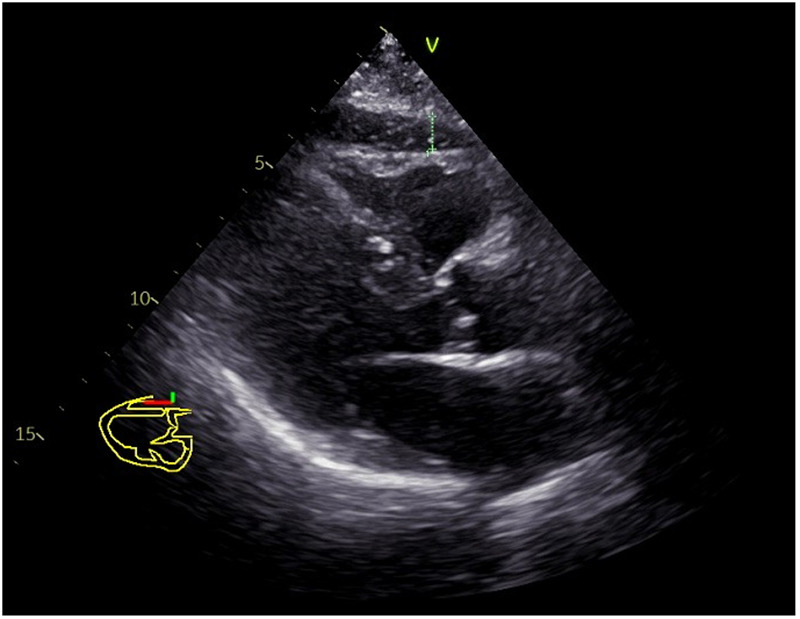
Transthoracic ultrasound measurement of EAT.
